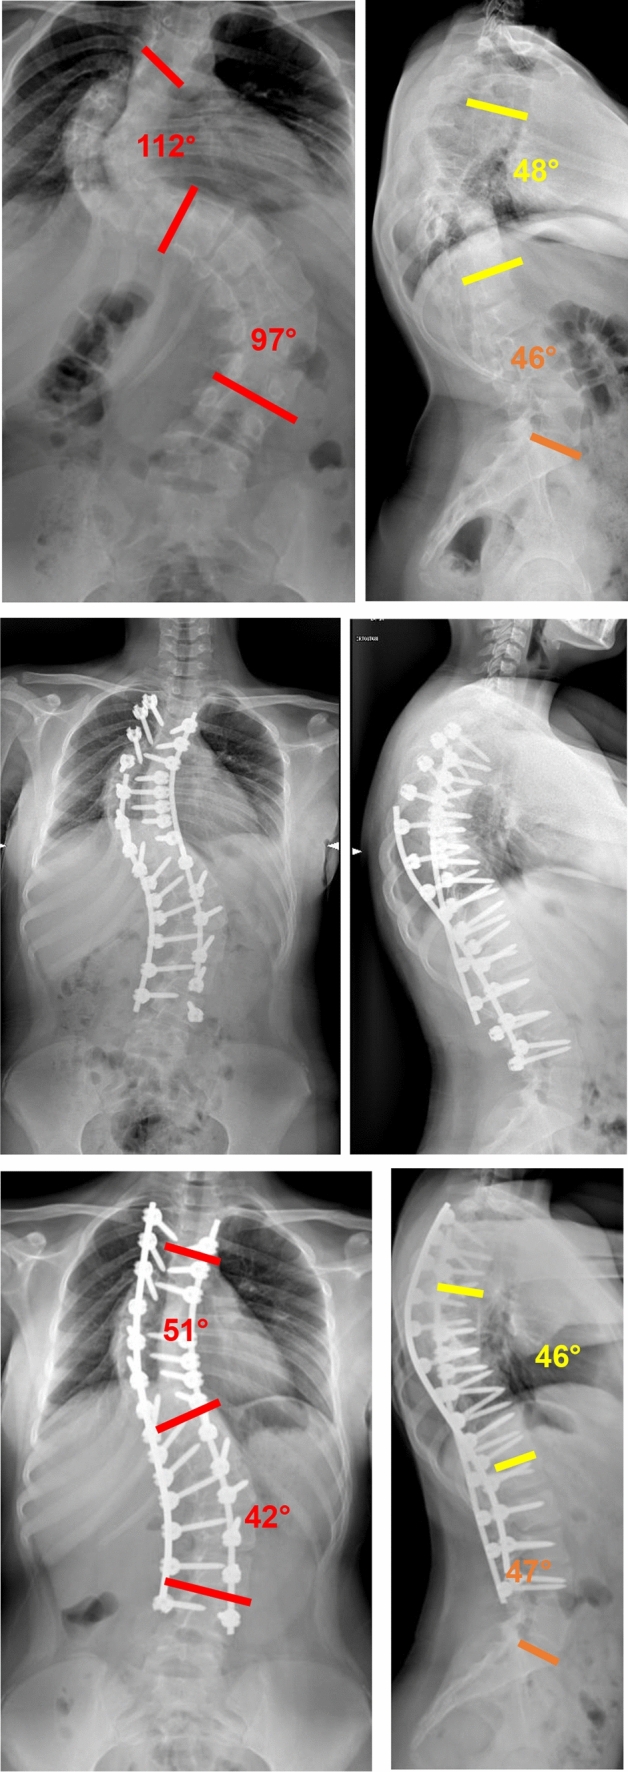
Case 2, a 15 years old female with a Lenke 3 curve, treated by T3–L4 fusion. The patient had a transient SEP and MEP drop during corrective maneuver, so the patient was temporarily stabilized with two titanium rods and managed with a second stage surgery after 5 days. Pre-operative and post-operative (first and second stage surgery) X-rays
